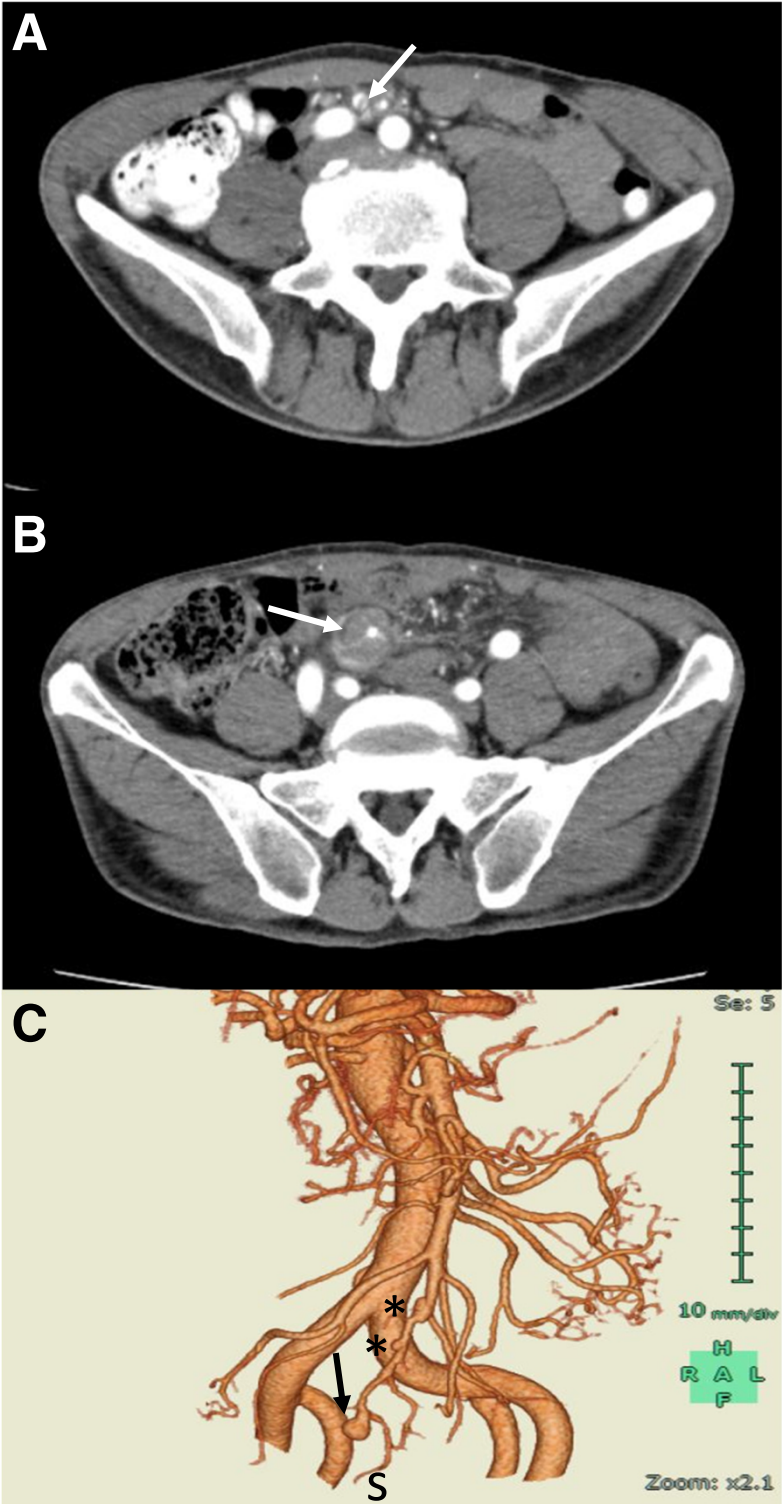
Contrast-enhanced CT images on admission. a
